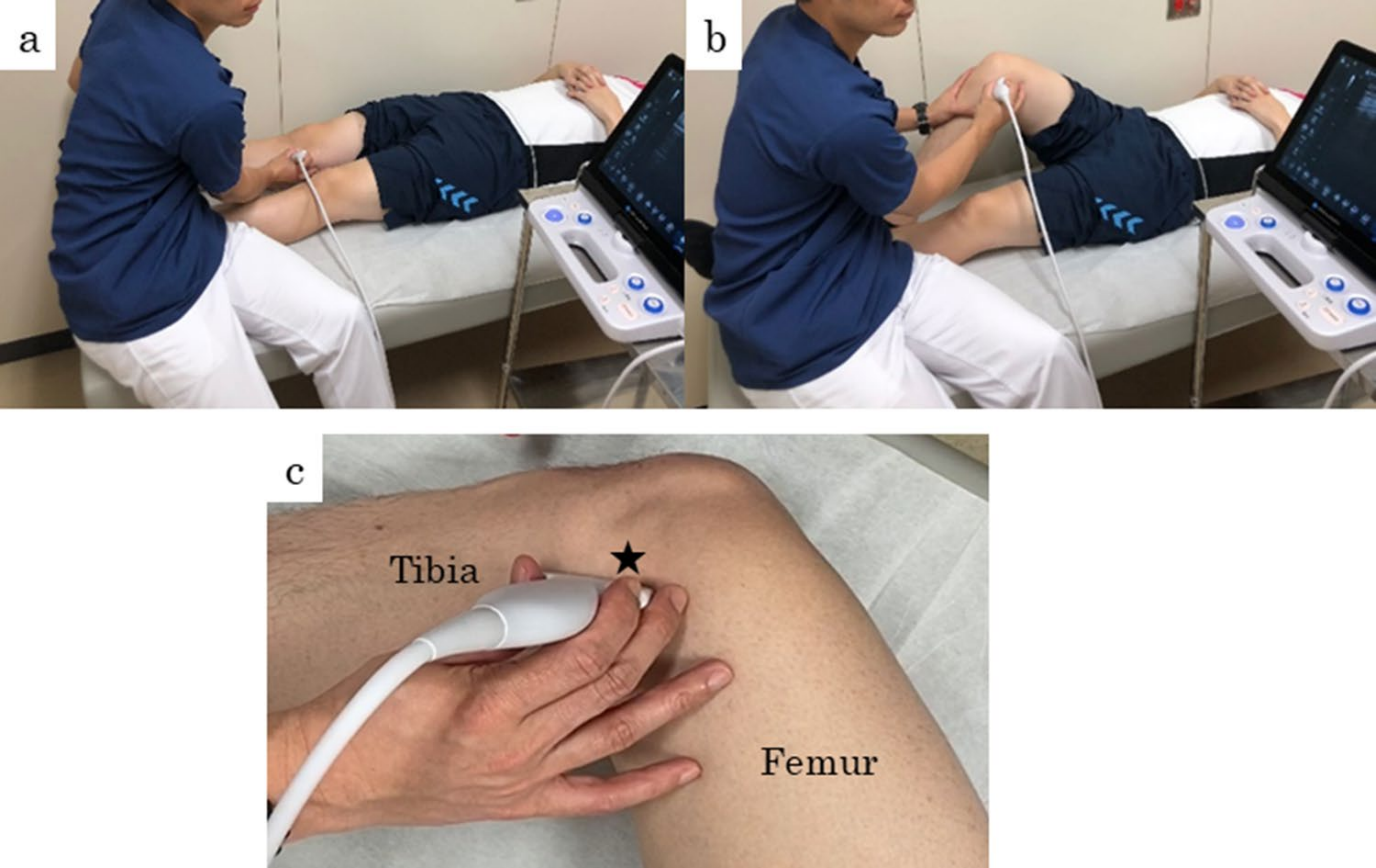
Ultrasonographic evaluations in the supine position at knee flexion angles of (a) 0° and (b) 90°. The appropriate probe position during MME evaluation (c). The femoral medial epicondyle was designated as a bony landmark (asterisk), and the proximal part of the probe was installed just behind it. *MME* medial meniscal extrusion.
